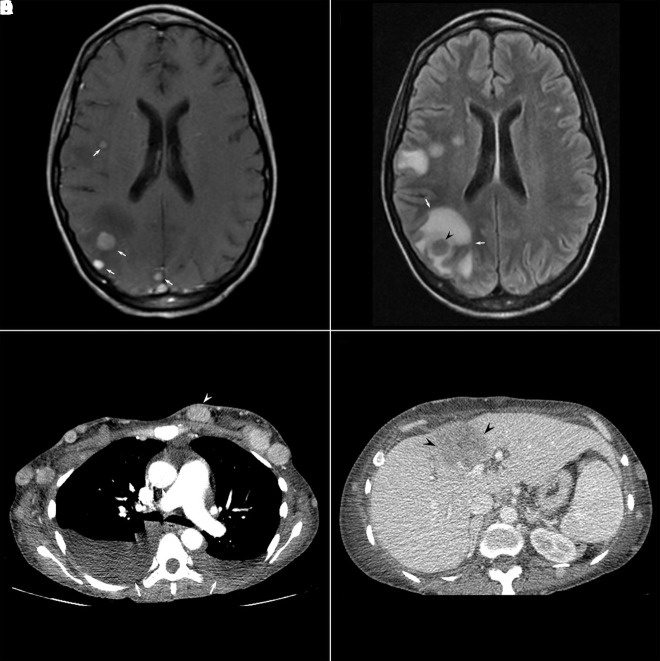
Radiology findings. (A) Axial T1 weighted image with contrast demonstrates multiple enhancing intracranial lesions (white arrows) within the right frontal and parietal lobe consistent with metastases. (B) Axial fluid-attenuated inversion recovery (FLAIR) images demonstrate varying degrees of vasogenic edema (white arrows) surrounding the brain metastases, most notably the right parietal lesion (black arrowhead). (C) Axial CT image of the chest demonstrates multiple subcutaneous metastatic nodules (white arrowhead on a representative nodule). Bilateral pleural effusions (white arrows) are also noted. (D) Axial CT image of the abdomen with contrast demonstrates the large hypoenhancing left hepatic lobe lesion, compatible with a hepatic metastasis (black arrowheads).
